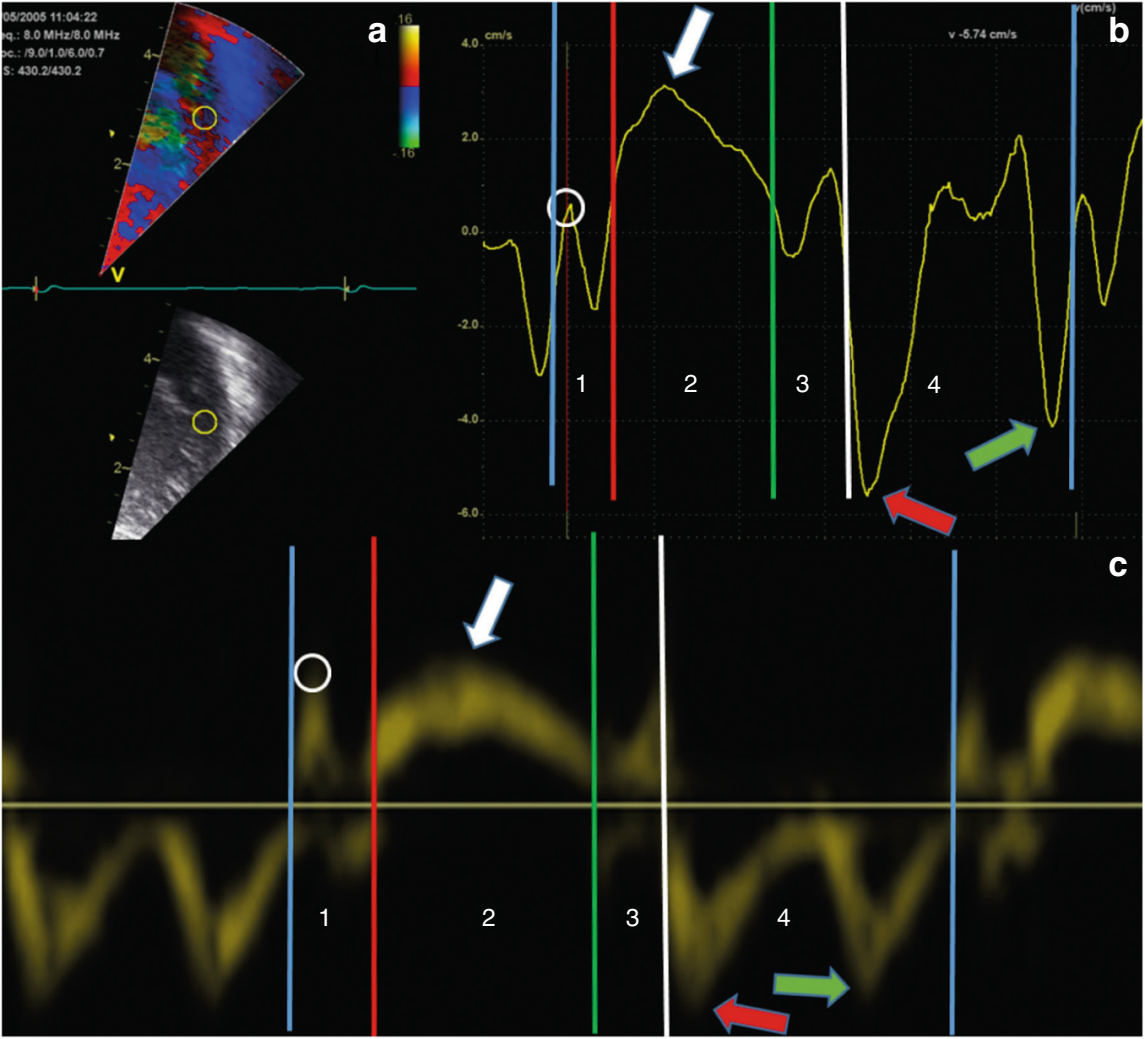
The upper panels show color-coded tissue Doppler velocity curve from a term neonate, from the left lateral atrioventricular plane from the apical four-chamber view. The upper left panel a shows the echocardiographic image with a sample area of 3 mm diameter (yellow circle). The curve in the upper right panel b shows the tissue velocities from the sample area. The lower panel c shows pulsed-wave tissue Doppler velocity curve from the septal hinge atrioventricular plane from the apical four-chamber view of a term neonate. White arrows at the peak systolic velocity (s′), red arrows at the early diastolic velocity (e′), and green arrows at the peak velocity in atrial systole (a′). The vertical lines denote the events defining the four phases of the heart cycle; isovolumic contraction,^1^ ejection phase,^2^ isovolumic relaxation,^3^ and filling.^4^ Note that the velocity curves in the isovolumic phases (1 and 3) contain one negative peak adjacent to the ejection phase^2^ and one positive peak adjacent to the filling phase,^4^ most evident in the color-coded measurements. Blue vertical lines denote closure of the mitral valve. Red vertical line denotes opening of the aortic valve. Green vertical line denotes closure of the aortic valve. White vertical line denotes opening of the mitral valve. White circles denote the peak isovolumic contraction velocity. *X*-axis: time. *Y*-axis: tissue velocity from the sample area
